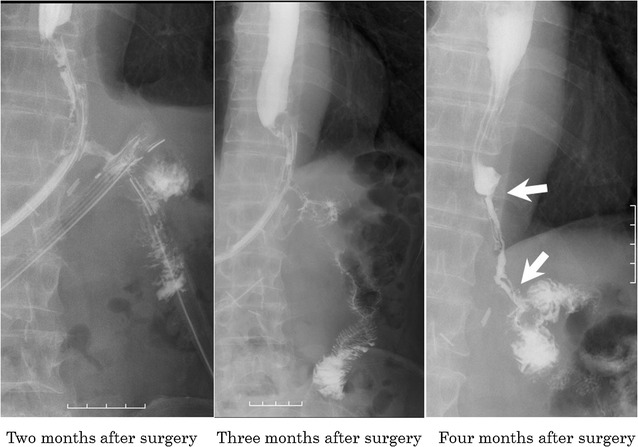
Oral contrast radiography revealed a fistula. Contrast medium was observed leaking from the dehiscence into the drainage tube after 2 and 3 months, but this had stopped by 4 months postoperatively, and the contrast medium was observed to flow from the esophagus to the jejunum, suggesting that a fistula had formed between the two ends
